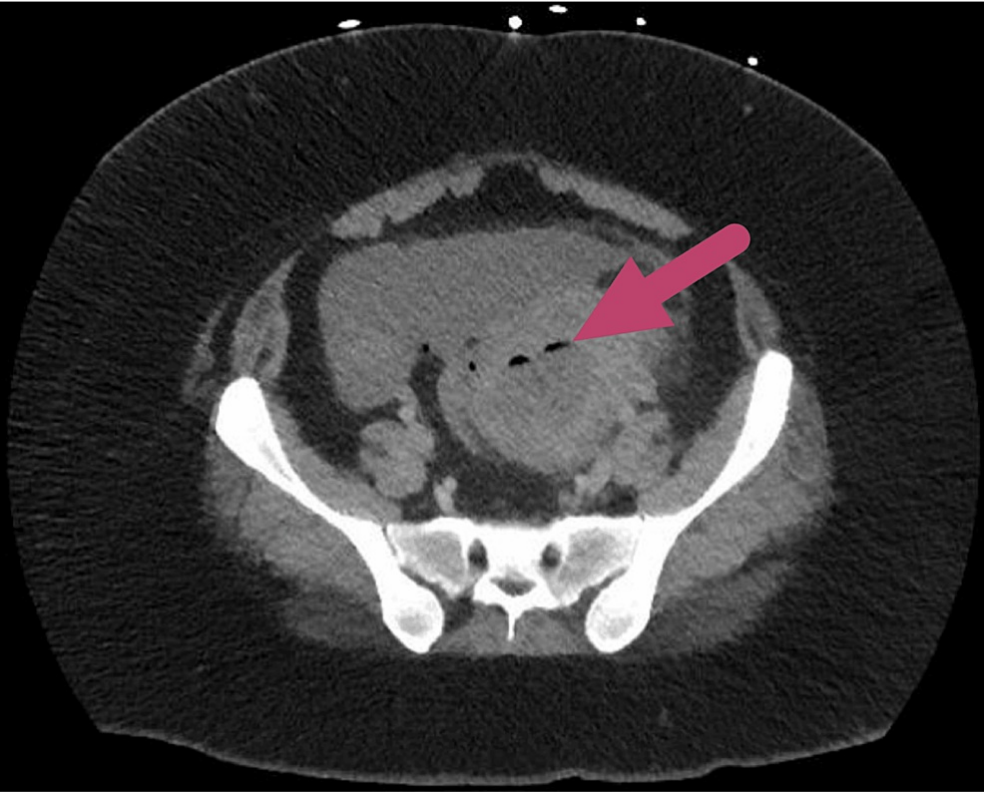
Computed tomography showing the left adnexal mass with internal air (red arrow).
